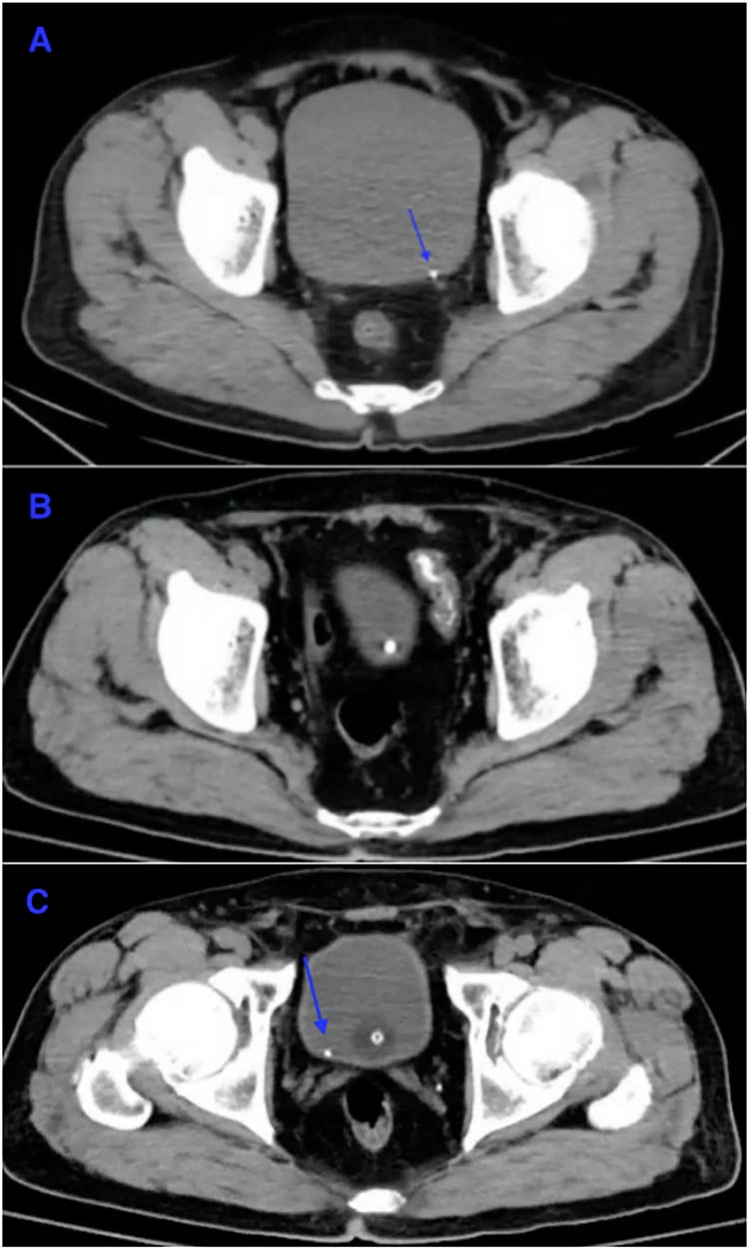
Non-contrast CT scan of the abdomen and pelvis. Axial slice. (A) On admission, there was a 4 mm left distal ureteric stone (arrow) is present near vesicoureteric junction. (B) On POD10, the left distal ureteric stone has passed. In-dwelling urinary catheter was in situ. (C) The left ureteric stone has descended into the bladder (arrow).
